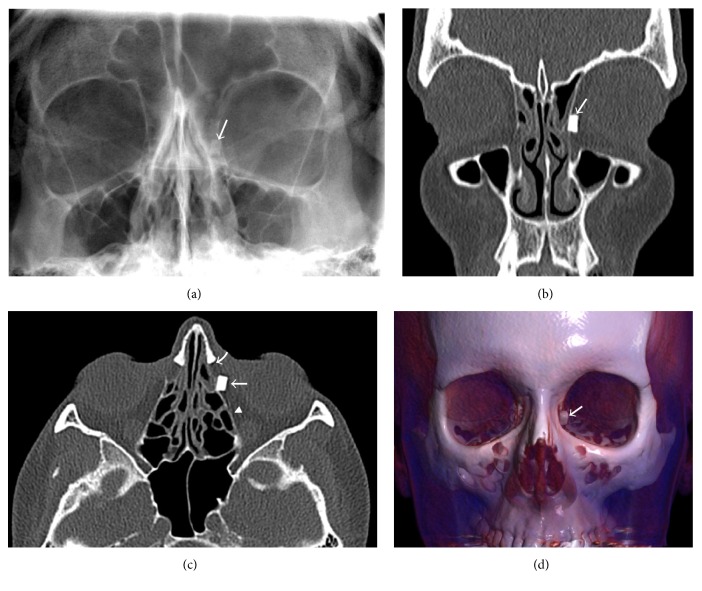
(a–d) Frontal plain radiograph revealing (a) a rectangular high-density body (arrow) overlying the left ethmoid cells and in contact with the lamina papyracea. Contrast unenhanced axial (b) and coronal (c) CT scans show a well-delineated hyperdense body (arrow) within the left orbit, adjacent to the lamina papyracea (arrowhead in (c)) and immediately posterior to the lacrimonasal duct (curved arrow in (c)). (d) 3D-CT volume-rendered reconstructed image shows the intraorbital metallic foreign body (arrow).
